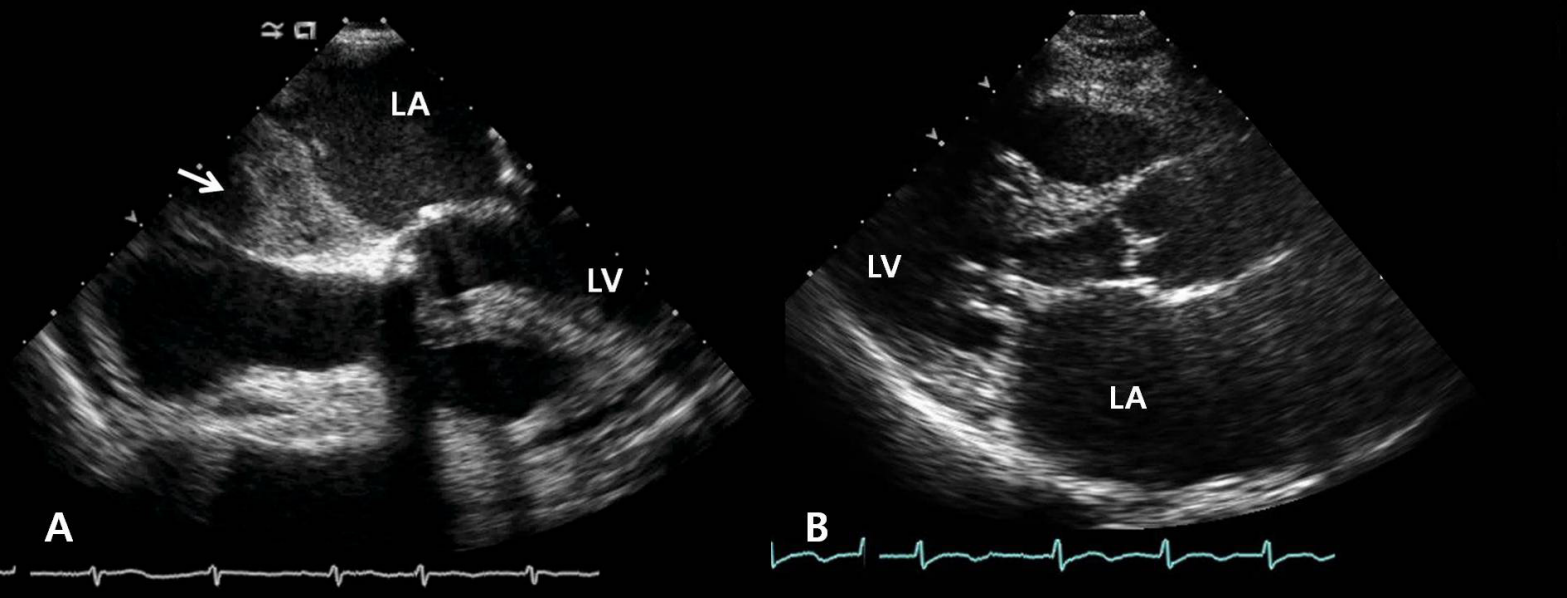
A) Transesophageal echocardiogram shows new thrombus formation in the left atrium (arrow). B) Left atrial thrombus was not seen in transthoracic echocardiogram (postoperative 3 months). LA: left atrium; LV: left ventricle.
